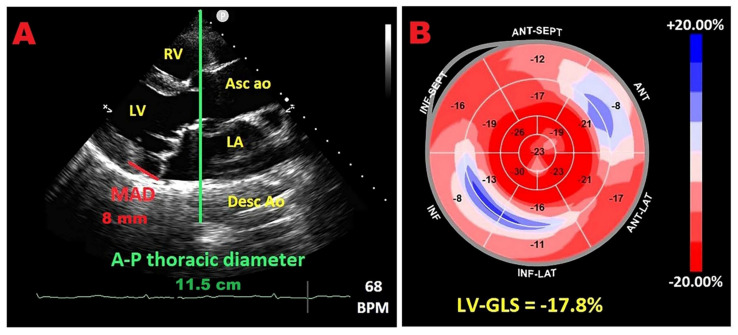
Representative example of an MVP individual with MAD, narrow A-P thoracic diameter, and mild deterioration of LV–GLS with “apical sparing” pattern. (A) Parasternal long-axis view showing MVP, MAD, and reduced A-P diameter (red line indicates MAD distance; green line indicates A-P thoracic diameter). (B) 2D–STE bull’s eye plot depicting mild LV–GLS reduction with predominant basal involvement and relative apical sparing. Ao, aorta; A-P, antero-posterior; Asc, ascending; Desc, descending; GLS, global longitudinal strain; LV, left ventricle; MAD, mitral annular disjunction; MVP, mitral valve prolapse; RV, right ventricle.
